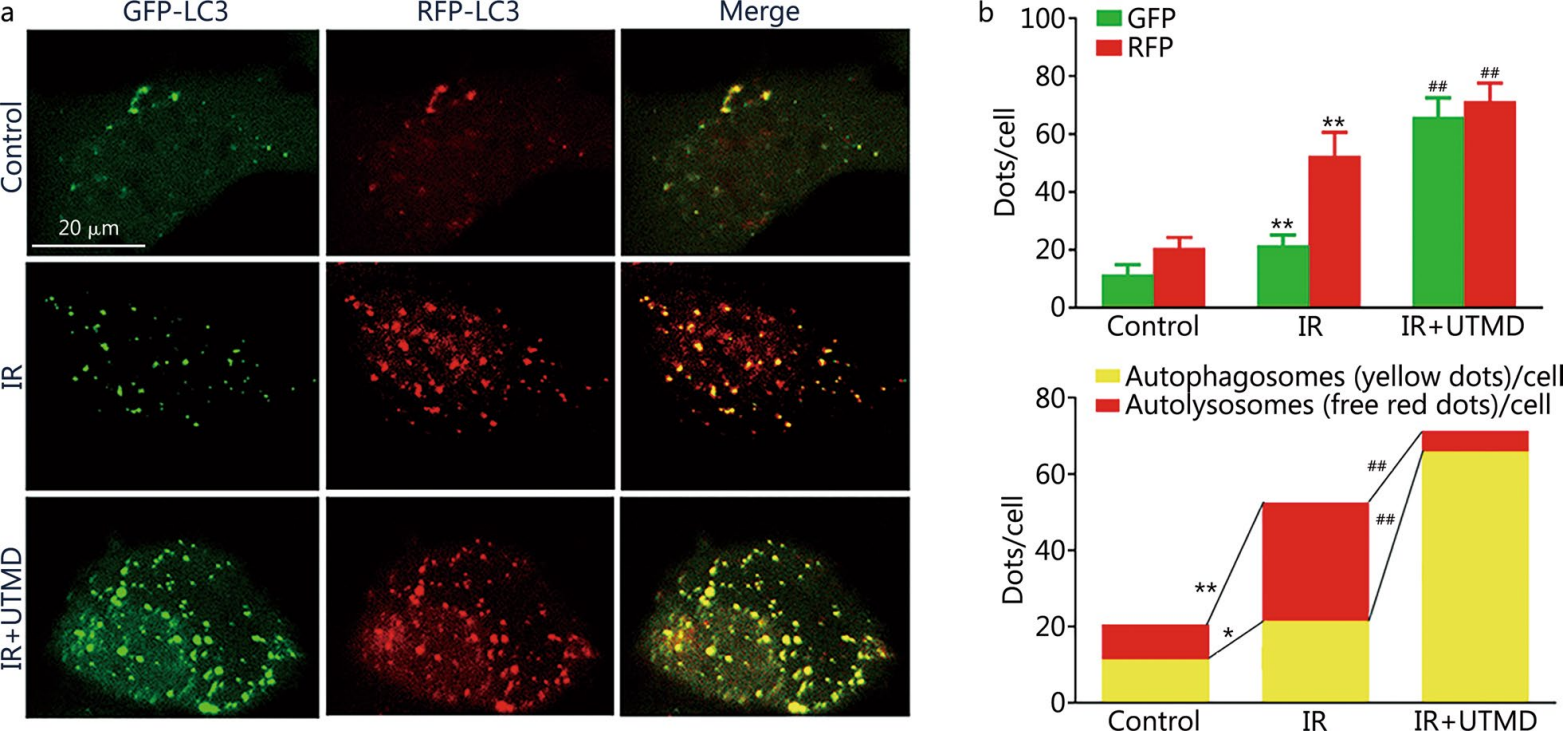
UTMD inhibited autophagosome fusion with lysosomes in IR-treated glioblastoma cells. GL261 cells were transfected with RFP-GFP-LC3B for 24 h and then exposed to UTMD (ultrasonic intensity of 1.2 W/cm^2^ combined with 200 μl/ml MBs at a duty cycle of 10% for 60 s) followed by IR (2 Gy) exposure. a Representative images of fluorescent LC3 puncta are shown. b Mean numbers of GFP and RFP dots/cell (top). Mean number of autophagosomes (yellow dots in merged images) and autolysosomes (red dots in merged images)/cell (bottom). Values are expressed as mean ± SD (*n* = 3). P* < 0.05, *P* < 0.01 vs. the vehicle-treated control group; ^##^*P* < 0.01 vs. IR group. GFP green fluorescent protein, RFP red fluorescent protein, LC3B light chain 3 beta, IR ionizing radiation, UTMD ultrasound-triggered microbubble destruction, MBs microbubbles, SD standard deviation
